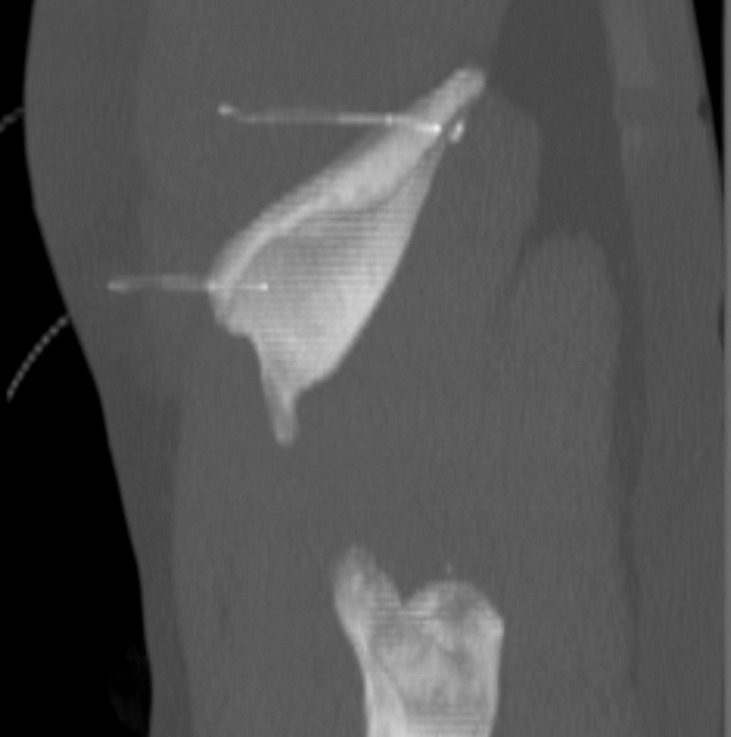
pre-operative targeting of the metastasis using hook wires placed, under CT-guidance, on both sides of the lesion
